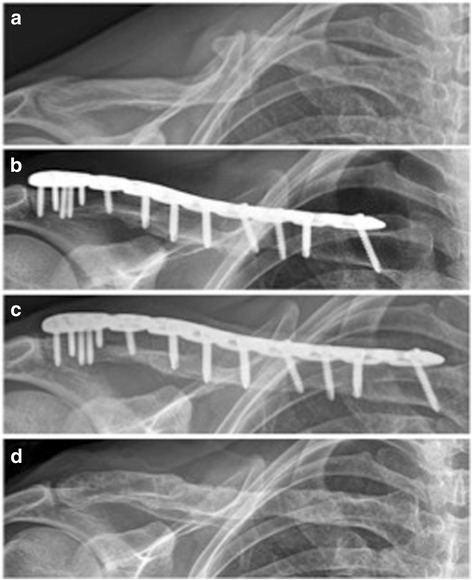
Radiographical outcome of a clavicular non-union (patient No. 10). a preoperative; b postoperative; c 2-year follow-up; d after plate removal
